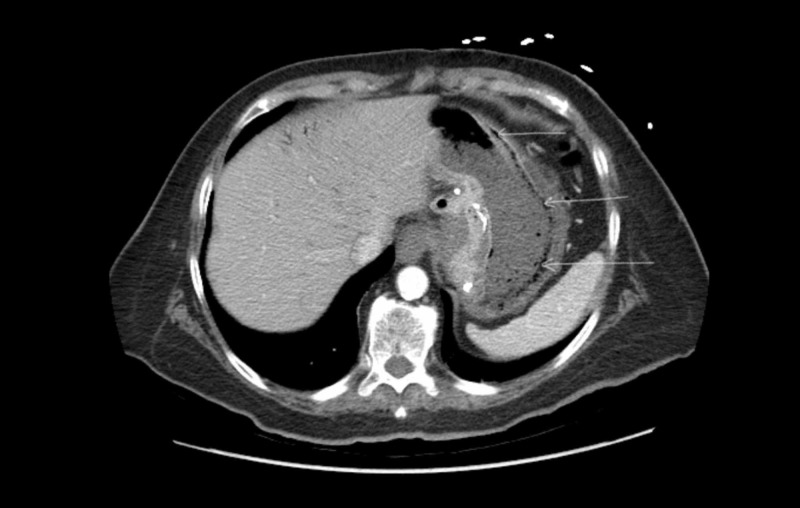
Computed tomography (CT) of the abdomen (different slice) showing gastric emphysema (arrows)
